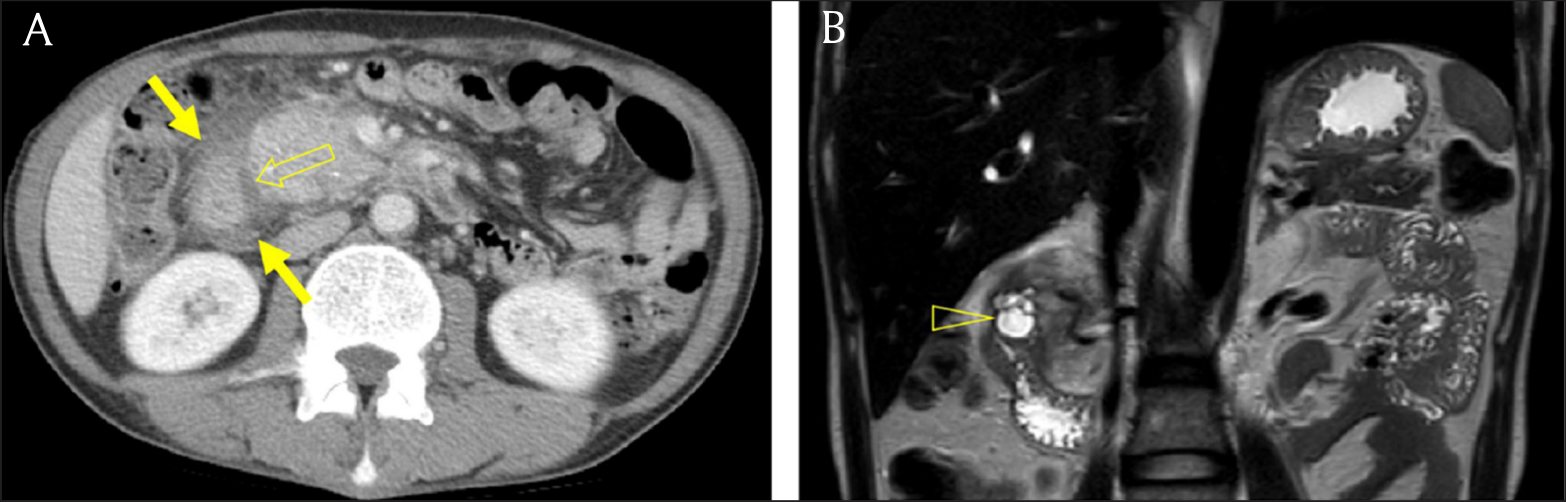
A 49-year-old man with paraduodenal pancreatitis. (A) Axial portal venous phase CT image shows fat infiltration and fluid collection (arrows) around the pancreaticoduodenal groove (open arrow). (B) Coronal T2-weighted image shows cystic lesion (open arrowhead) within the second portion of duodenum, suggesting cystic dystrophy of heterotopic pancreas.
